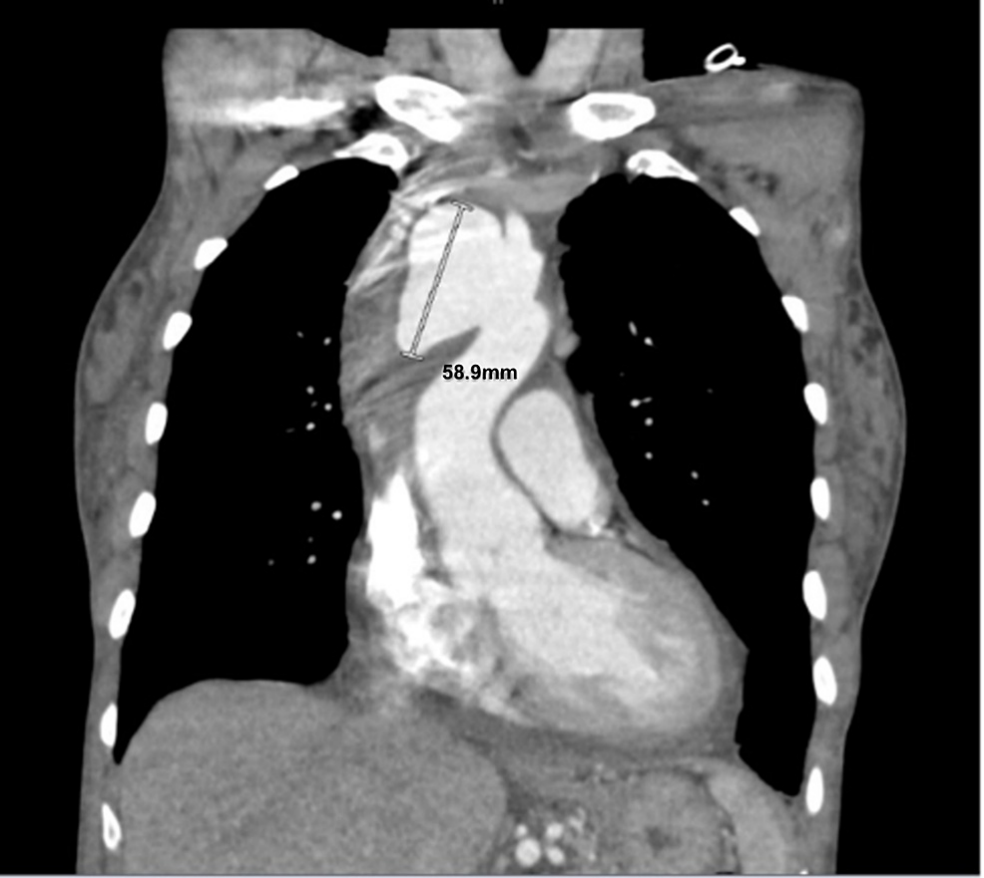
CT thorax demonstrating innominate artery aneurysm
